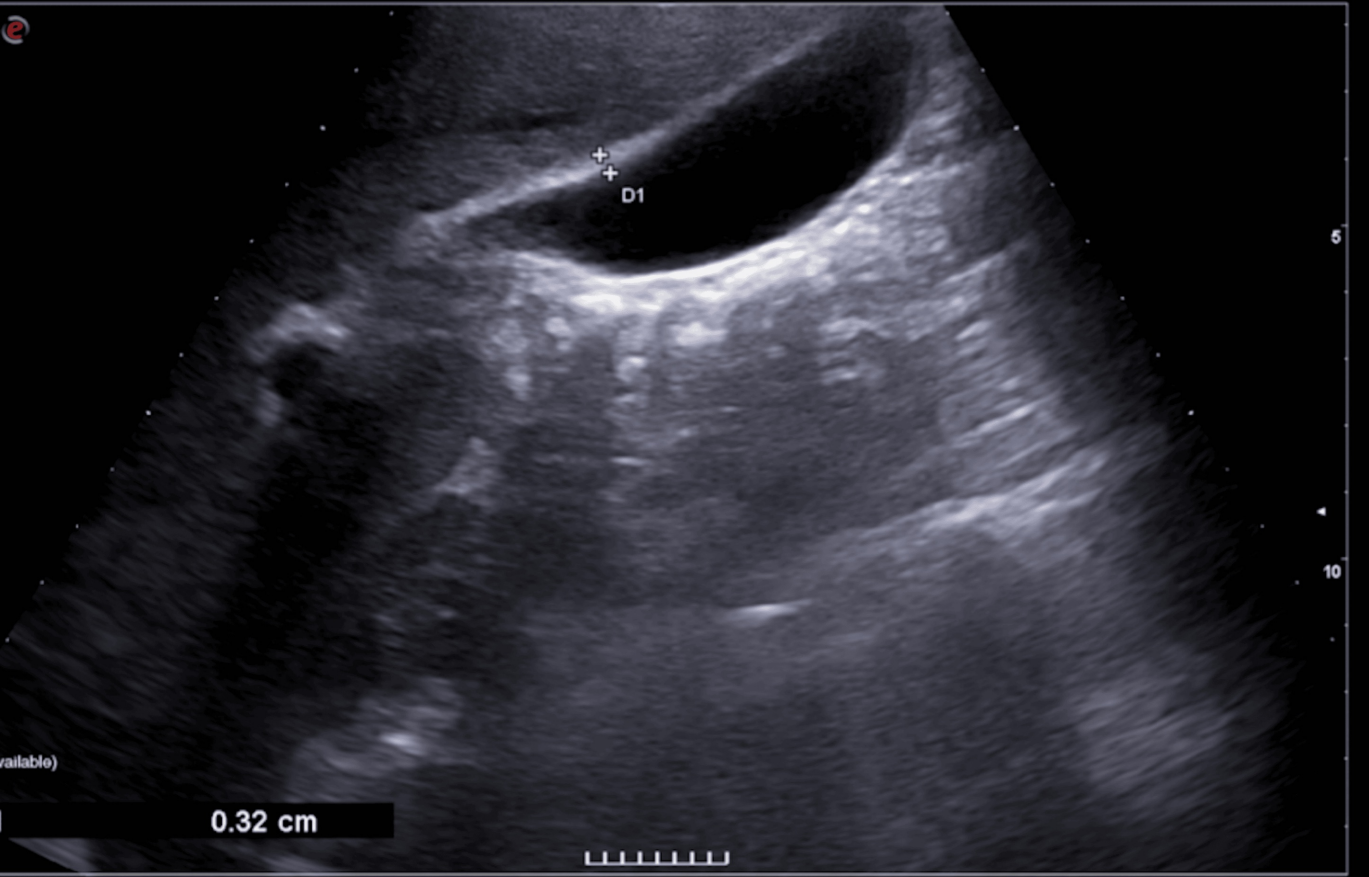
Ultrasonographic image of the gallbladder during the initial emergency department visit, which demonstrates a gallbladder with normal wall thickness (3.2 mm)
